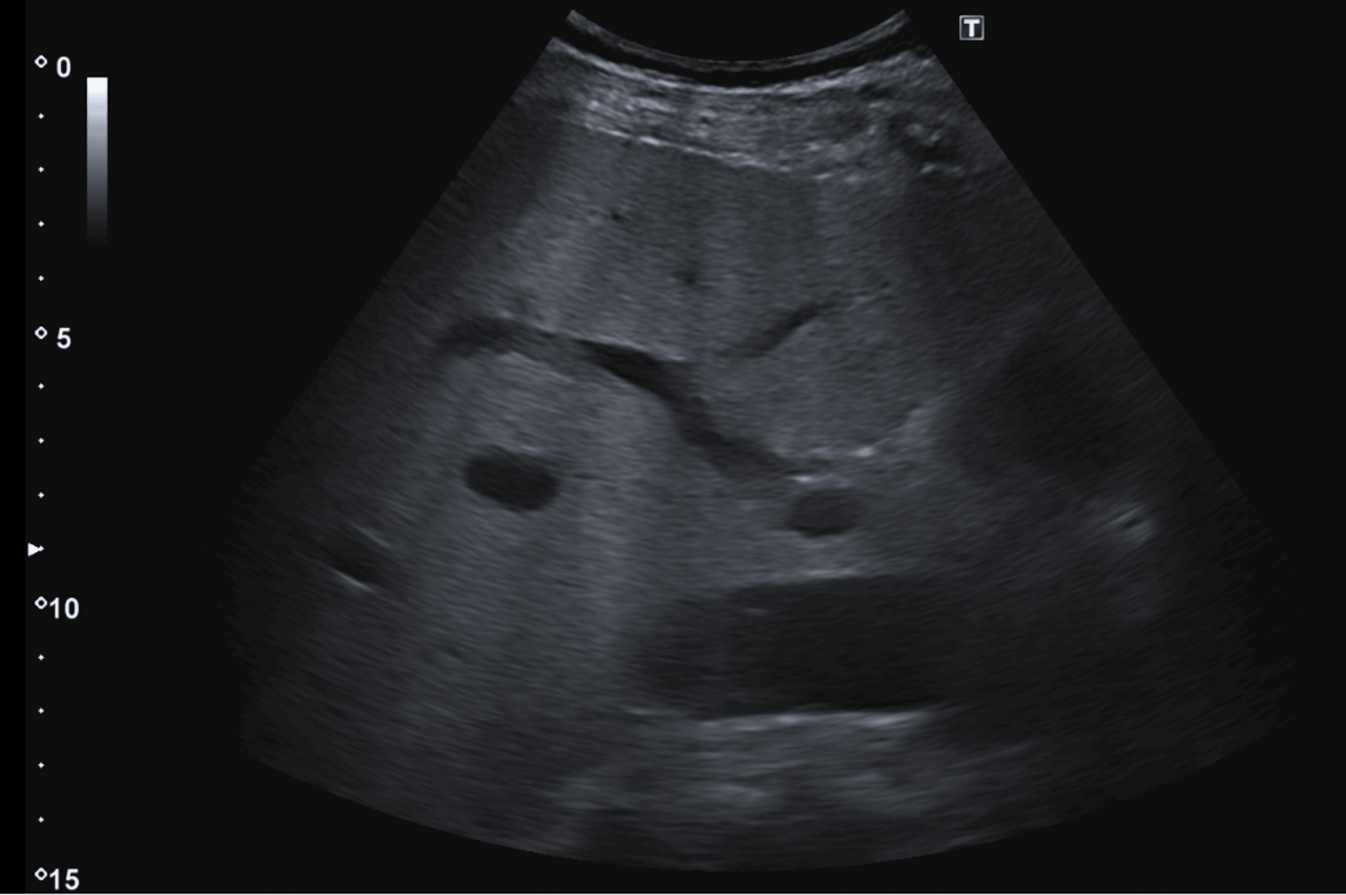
Abdominal ultrasound Abdominal ultrasound report highlights a large homogenous but hyperechoic liver, correlating to chronic liver disease.
